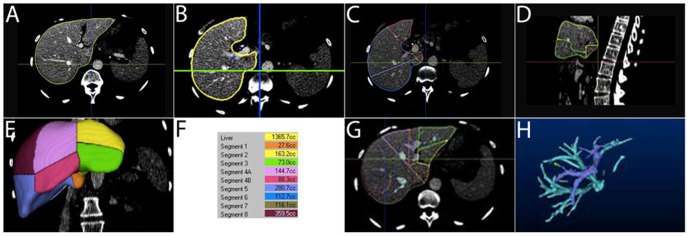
Semi-automated Interactive Software (P) – Image Example. A Transverse image of the portal-venous phase – automated outline of the entire liver after manual correction of false-positive and false-negative extractions. B Manual positioning of the anatomical landmark “first bifurcation of the right portal vein” (blue circle) according to Fig. 1A. C Automated definition of segments of Couinaud for right liver - transverse image. D Automated definition of segments of Couinaud for left liver - sagittal image. E Volume rendering (coronal view) with automated definition of segments of Couinaud of the entire liver. F List of volumes for the different segments of Couinaud. G Transverse image of the portal-venous phase – automated outline of the entire liver after manual correction of false-positive and false-negative extractions. H Volume rendering (coronal view) with automated definition of vessels (liver veins in light blue and portal veins in dark blue). Note: in each live liver donor, CT-volumetry of the entire liver was performed to ensure that the postoperative liver volume, calculated on the basis of Fig. 5F, is adequate.
